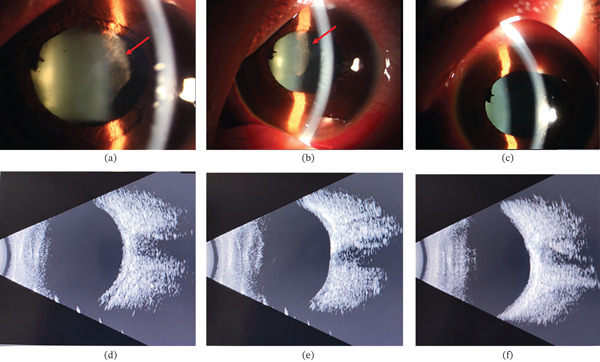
Sequential anterior segment photographs (top row) and corresponding ocular ultrasound images (bottom row) of the left eye during hospitalization. (a, d) Day 2: Partial clearance of anterior chamber opacities is observed (red arrow), with residual echoes visible on ultrasound. (b, e) Day 3: Marked resolution of anterior chamber clouding with further normalization of ultrasound findings. (c, f) Day 6: The anterior chamber appears clear, and ultrasound confirms the absence of significant pathological changes.
